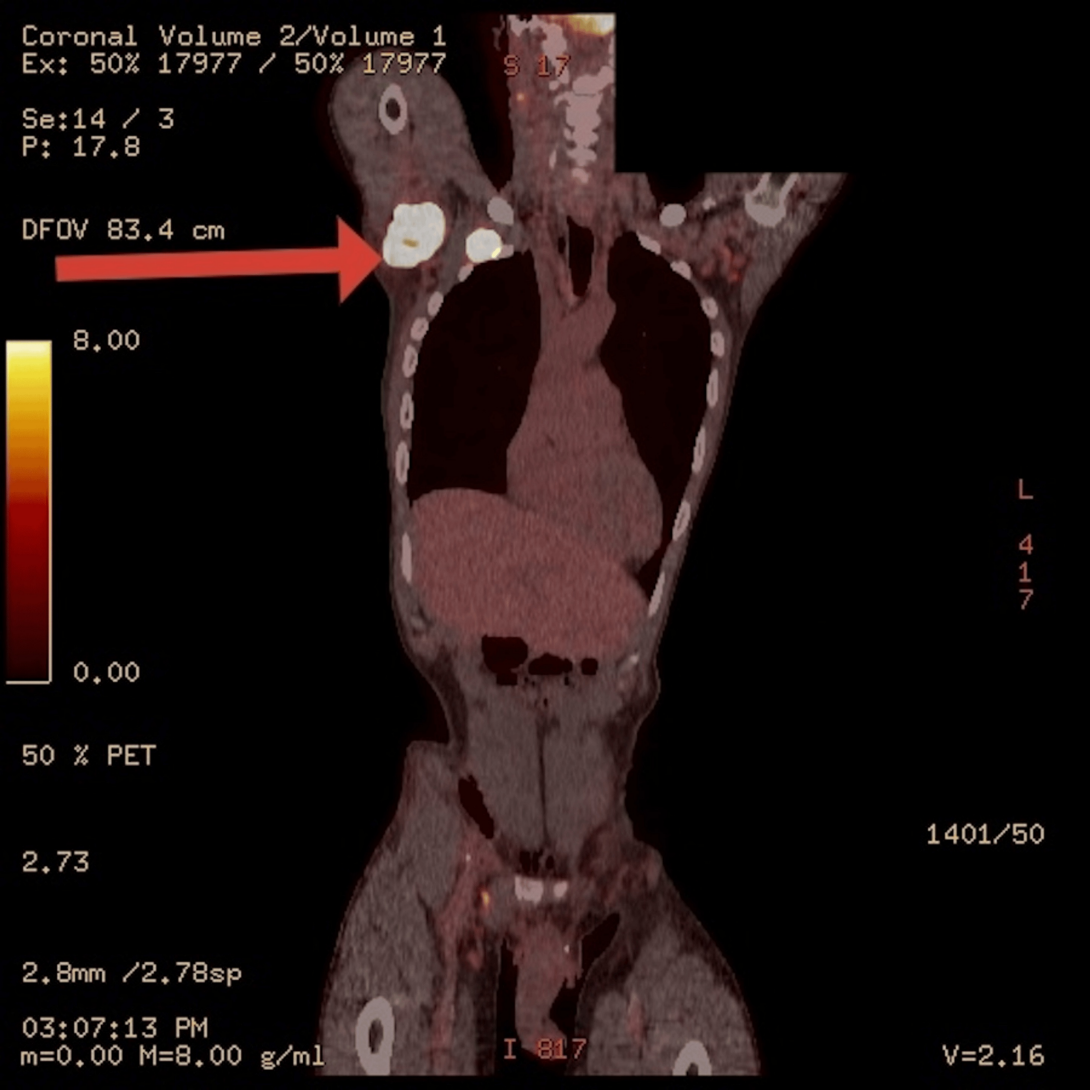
A PET-CT scan image demonstrating a metabolically active right axillary lymph node, as indicated by the red arrow PET - positron emission tomography
